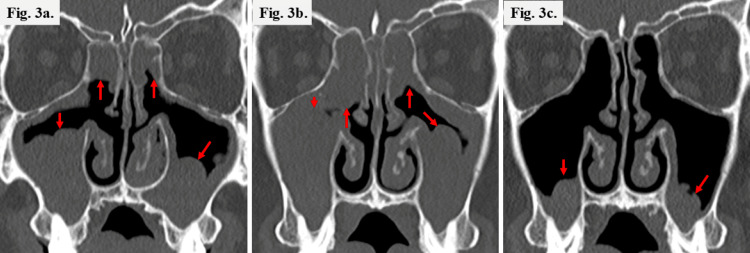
CT findings (a) before DUP administration, (b) five months after DUP administration, and (c) four months after TEZ administration a: soft tissue density is observed in the maxillary and ethmoid sinuses (red arrows); b: despite five months of DUP therapy, paranasal sinus opacification (red arrows) has further worsened; c: overall paranasal sinus opacification has resolved with only a faint residual shadow remaining on the floor of the maxillary sinus (red arrows). CT: computed tomography; DUP: dupilumab; TEZ: tezepelumab
